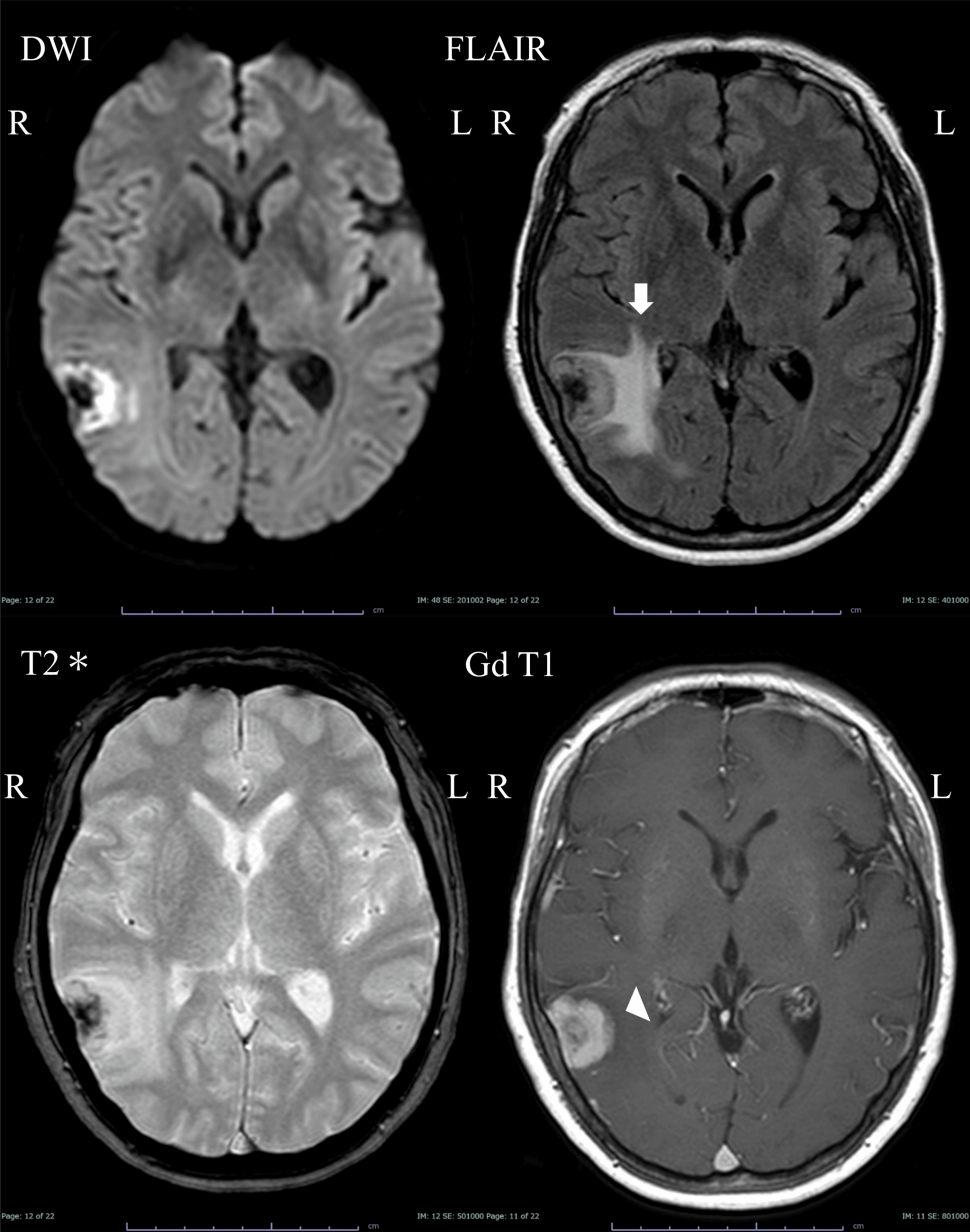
Initial magnetic resonance images. The middle cranial fossa is accompanied by perifocal cerebral edema (white arrow) with an extra-axial mass (white arrowhead). DWI: diffusion-weighted image, FLAIR: fluid-attenuated inversion recovery image, T2*: T2 star-weighted image, Gd T1: gadolinium contrast-enhanced T1-weighted image.
